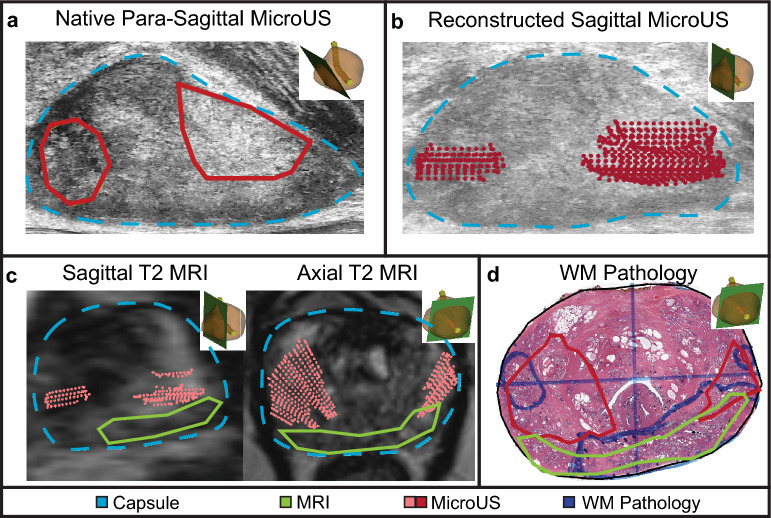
Complete registration process from microUS to WM pathology. (a) MicroUS reviewer delineations (red) are made on native microUS imaging. (b) These markups are turned into a point-cloud and positioned in 3D space similar to the image reconstruction. (c) The transformation matrices for microUS to MRI registration are applied to the markup. (d) Lastly, the microUS and MRI (green) delineations are co-registered with WM pathology.
